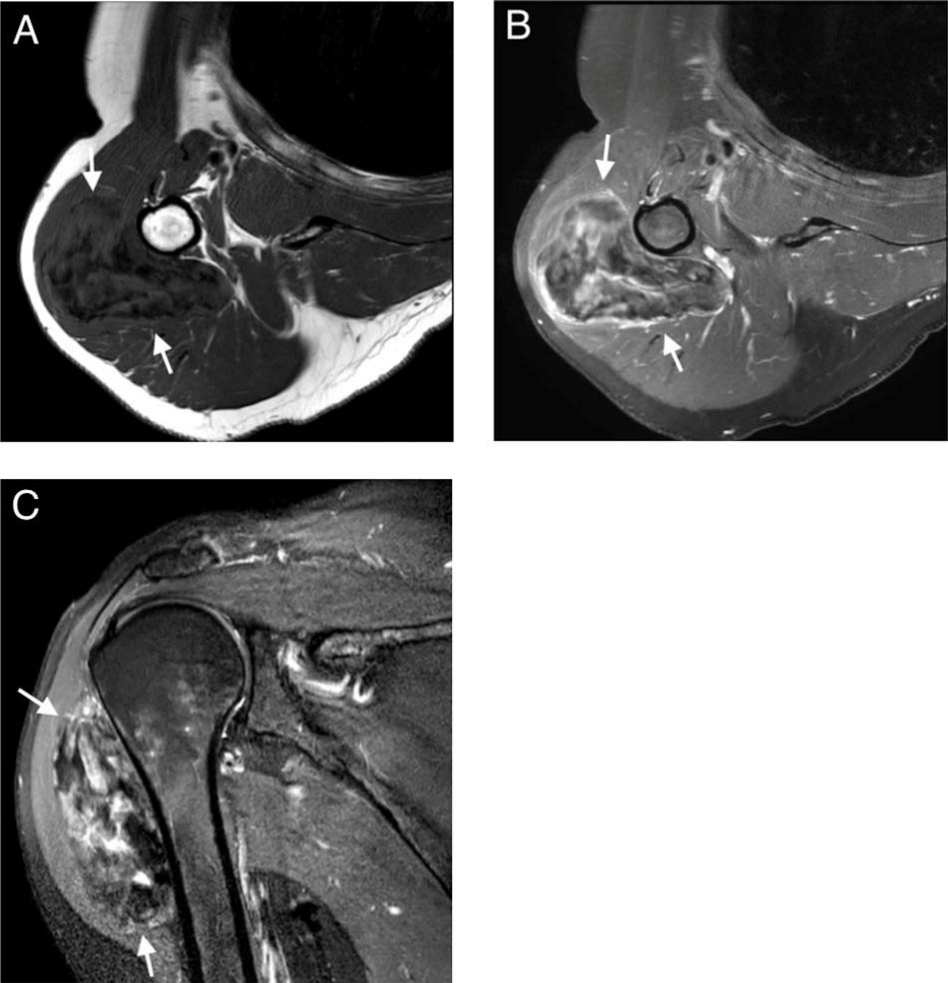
MRI with (A); axial T1-WI; (B) axial T1-FS-WI after administration of intravenous gadolinium contrast and (C) sagittal T2-FS-WI. Heterogeneous tumoral mass indicated with long arrows.
